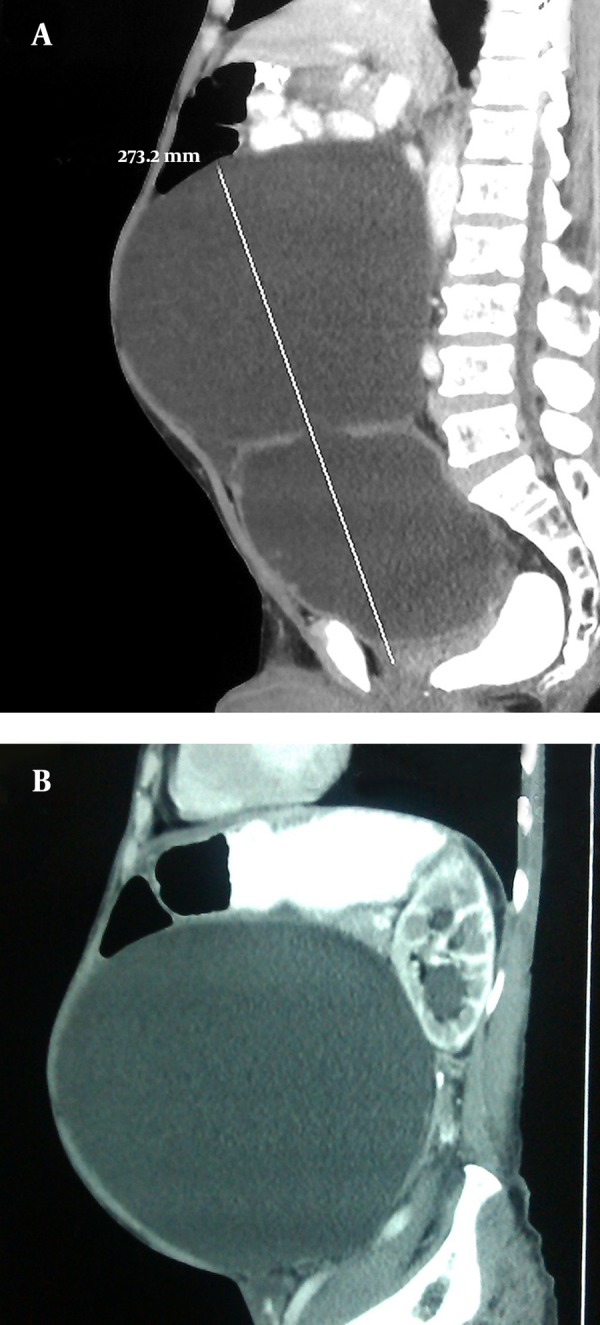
Contrast-Enhanced Computed Tomography of Abdomen Showing Giant Urinary Bladder Diverticulum
